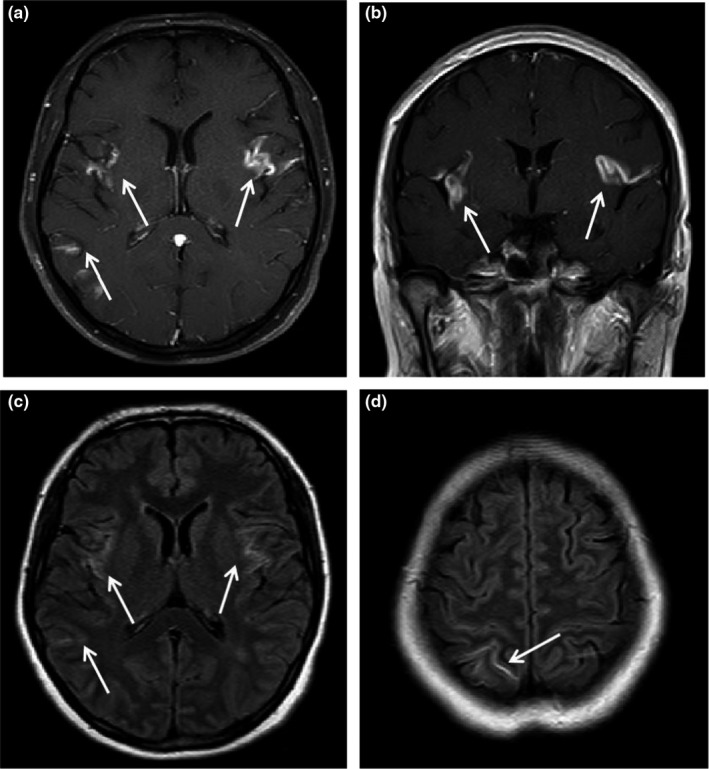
(a–d) A male, 35 years old. (a, b) Axial and coronal enhanced T1WI scans showing multiple long, abnormal enhancements in the pia matter. (c, d) Axial enhanced FLAIR image showing multiple long, abnormal enhancements in the pia matter, slightly lower than in the enhanced T1WI scan
